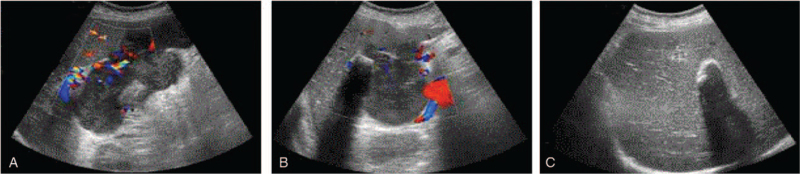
Abdominal ultrasound demonstrating enlarged gallbladder with destroyed structure (A), filled with hypoechoic mass (B) and gallbladder stones (C).
